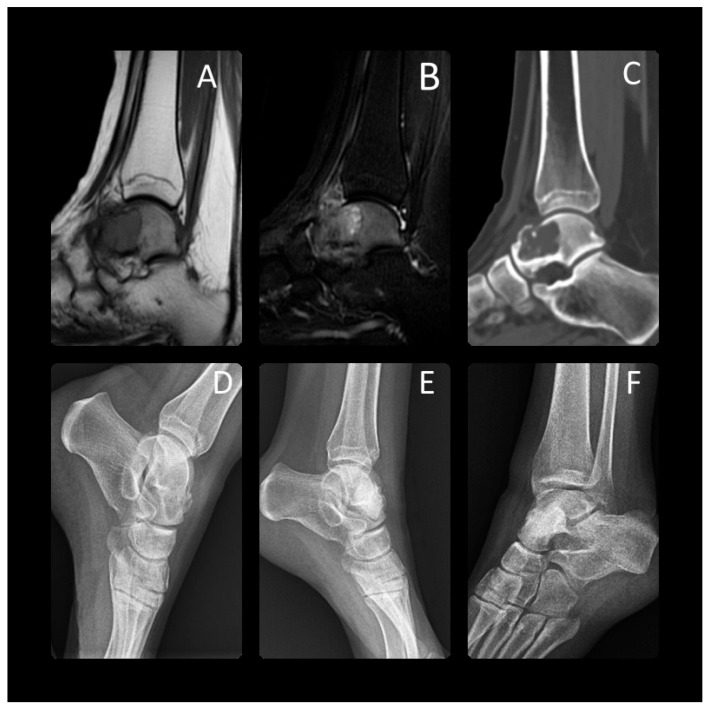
At the age of 17 years, this patient presented with chondroblastoma of the talus. A preoperative Tl-weighted (A) and T2-weighted (B) MRI, CT (C) and X-ray (D) radiograph showed a 3 cm lucent lesion within the talar neck. Expansile periosteal reaction was identified. In the distal part of the talus, particularly in the neck and the area near to the talonavicular joint, a microfracture was observed, along with considerable bone edema. This was treated with aggressive curettage and bone cementing. The final radiographs were taken 1 year (E) and 3 years (F) after surgery. Skeletal maturity shows the lesion had completely healed.
